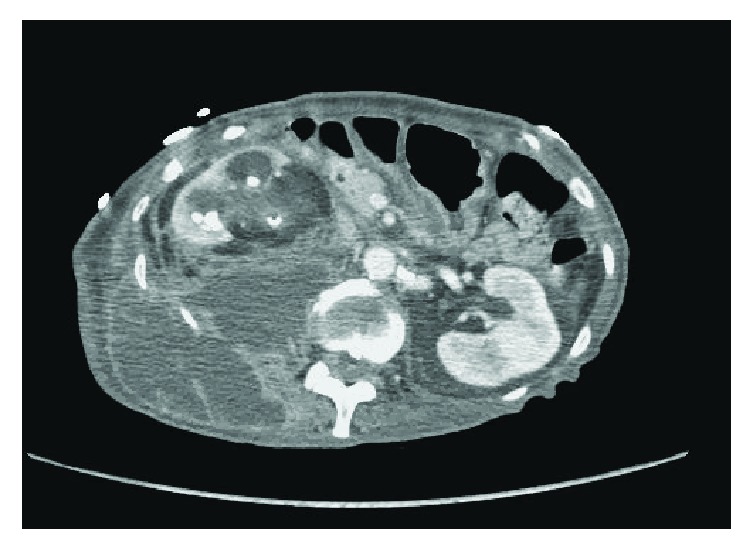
Axial section of a CT scan of the abdomen/pelvis with IV contrast demonstrating severe right hydronephrosis communicating with a large retroperitoneal fluid collection.
